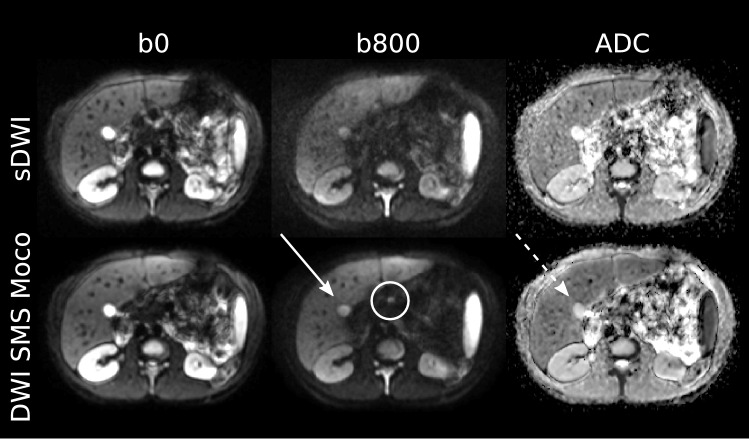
Comparison of image quality of sDWI and DWI SMS Moco in an 18-year-old patient with cystic fibrosis (CF), pancreas fibrosis and microgallbladder. Images show the acquired DWI images with *b*-values of 0 (b0) and 800 (b800) as well as the corresponding ADC map for both acquisitions. Shown is the sharp boundary of the microgallbladder in the b800 image (white arrow) of the DWI SMS Moco and the corresponding T2 shine-through effect in the ADC map (white dashed arrow). Further, a better differentiability of very small mesenteric lymph nodes in the DWI SMS Moco ventral to the thinned fatty pancreas in the b800 image can be observed (white circle)
